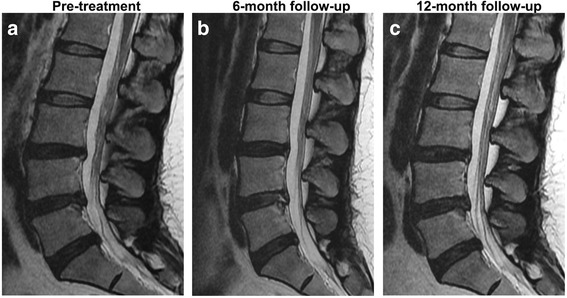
Assessment of disc degeneration grade using T2-weighted MRI. Sagittal T2-weighted images (a, pre treatment) show increased signal intensity of the L4/5 intervertebral disc from IV to III at 6 months (b) and 12 months (c), suggesting an increase of water content (case 1)
